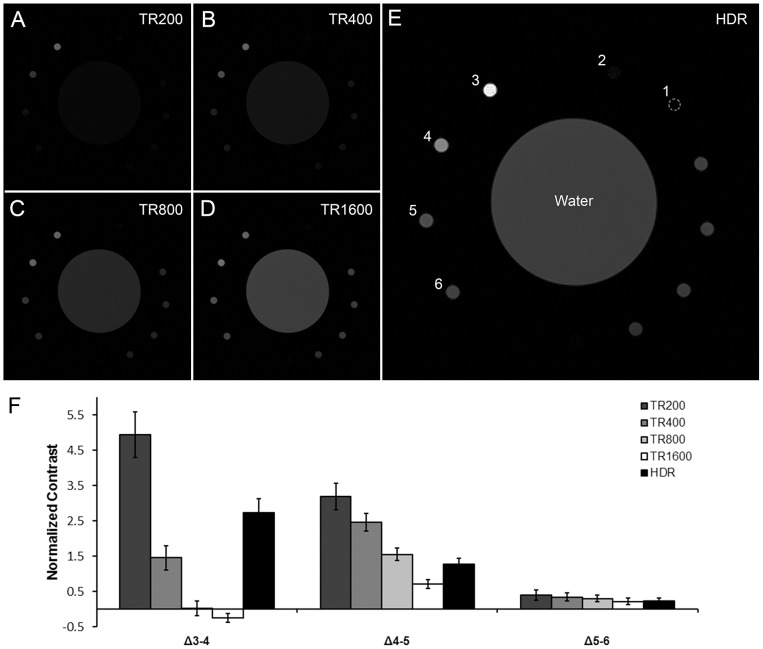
T_1_-weighted HDR-MRI. A series of images were acquired with constant T_E_ at 10.635 ms and varying T_R_ at (A) 200 ms (B) 400 ms (C) 800 ms and (D) 1600 ms. Images A–D were computationally merged by the HDR algorithm to generate the (E) HDR-MR image. The HDR image accentuated the contrast between samples 3 and 4 without suppressing samples 5 and 6 into the background. None of the LDR images, A–D, captured both features simultaneously. While image A had the largest normalized contrast, it also had the poorest SNR. Conversely, images with better SNR had worse normalized contrast, most notably between samples 3 and 4. HDR-MRI combined the complementary features of each image. (F) Quantification of contrast normalized against water. Error bars represent standard deviation.
